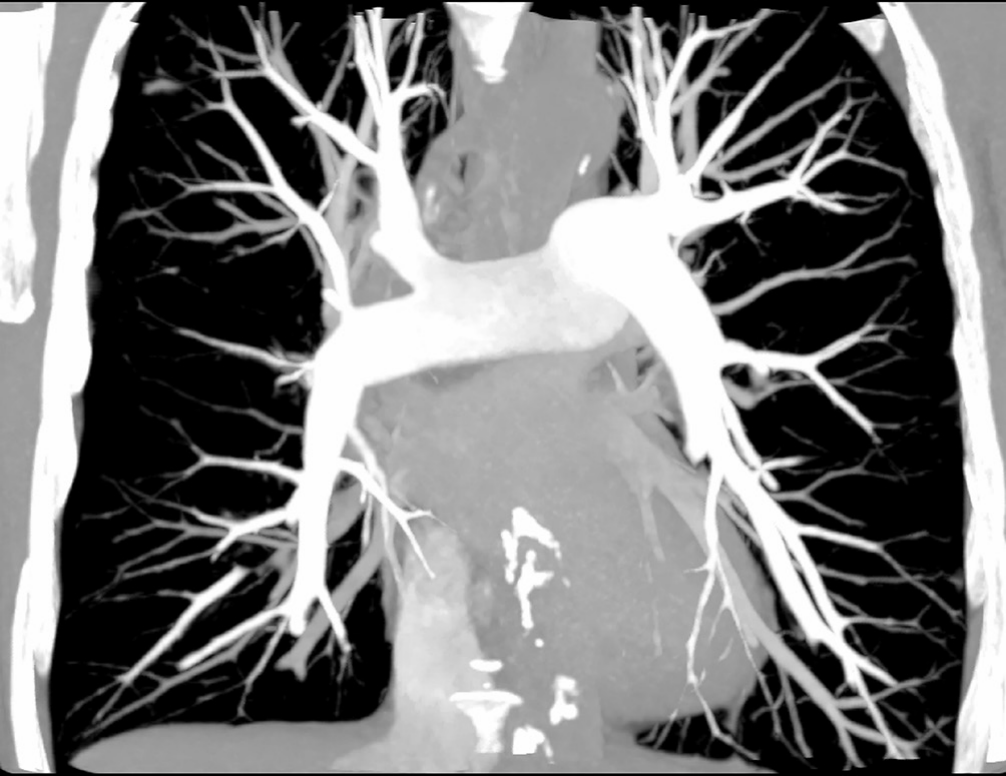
Coronal maximum intensity projection demonstrating the opacification of the pulmonary artery tree in a CTPA using 12 ml Iohexol 350 mg/ml in a 51-year-old woman. CTPA, CT pulmonaryangiography.
